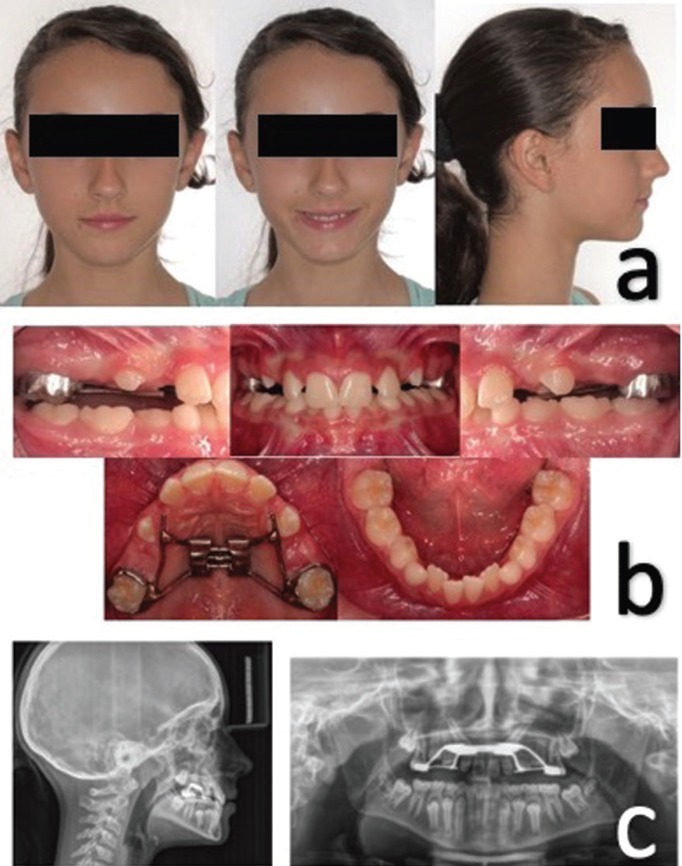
a) Extra oral clinical photographs pre-treatment. b) Intraoral photographs of the pre-treatment. c) Pre-treatment lateral and panoramic X-ray.
